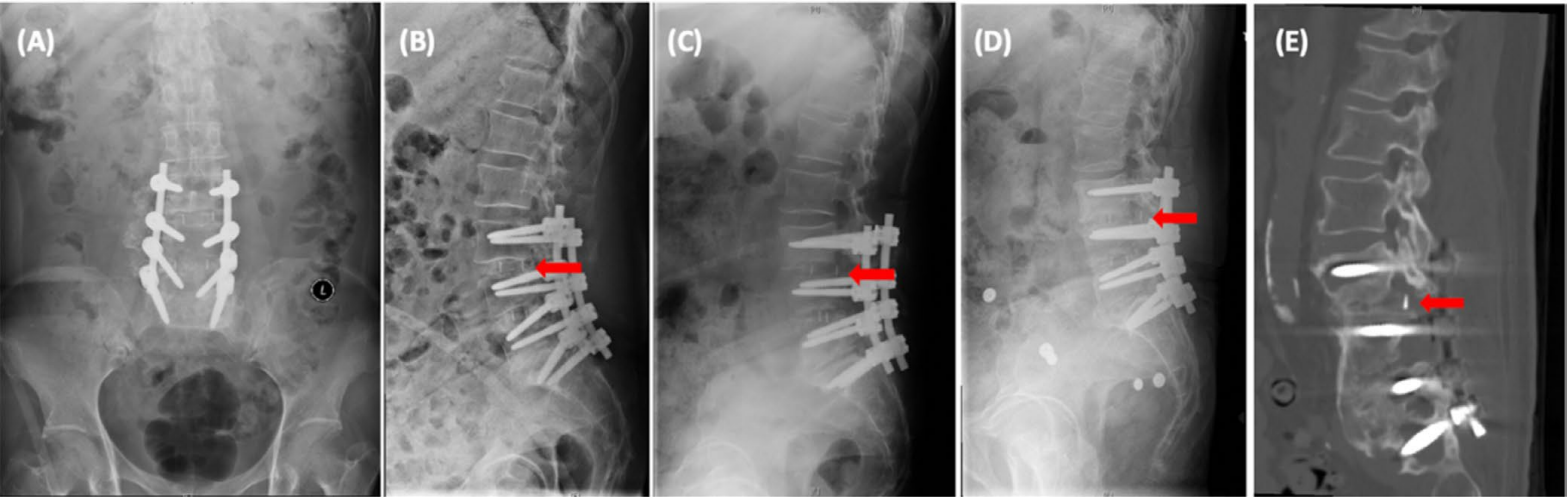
Images before and after conservative treatment for a female patient of 73 years old. (A) Postoperative anteroposterior radiogram. (B) Postoperative lateral radiogram. (C) The lateral radiogram at 3 months follow‐up shows the CR at L3/L4 level. (D) Lateral radiogram at 18 months follow‐up. (E) Sagittal reconstruction CT at L3/L4 level at final follow‐up, the patient's symptoms of lower back pain disappeared, and the CT indicated the successful fusion. The red arrow shows the posterior edge of the cage.
